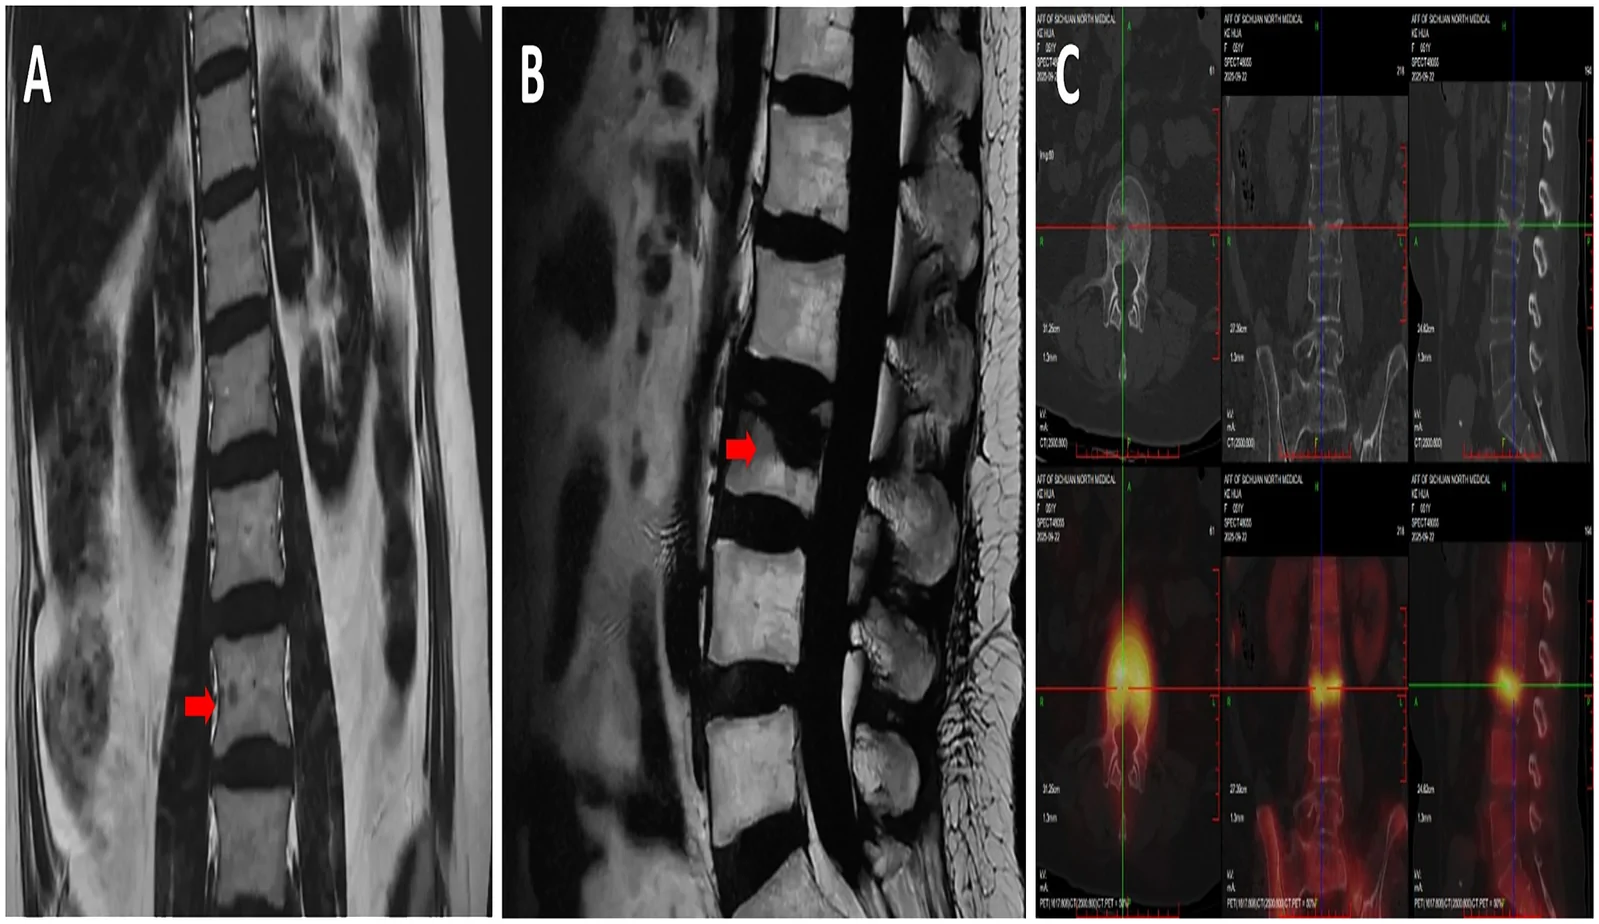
Representative imaging findings during treatment. (A) Lumbar spine MRI showed multiple enhancing nodules along the lumbar spine and in the submeningeal space. (B) Lumbar spine MRI showed a focal depression at the superior endplate of the L3 vertebral body. (C) SPECT-CT Image.SPECT-CT showed abnormal radiotracer uptake in the L3 vertebral body. Lesions are indicated by red arrows. MRI=magnetic resonance imaging. SPECT-CT, single photon emission computed tomography.
